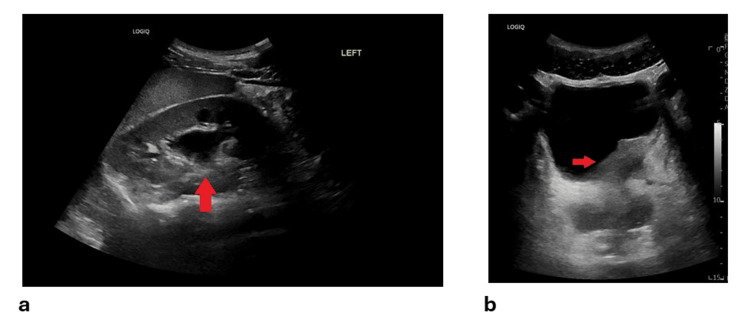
Ultrasonic images of the urinary tract. Ultrasound images showing (a) an arrow pointing to hydronephrosis of the left kidney, and (b) an arrow pointing to focal bladder wall thickening with a hypoechoic lesion around the left vesicoureteric junction.
